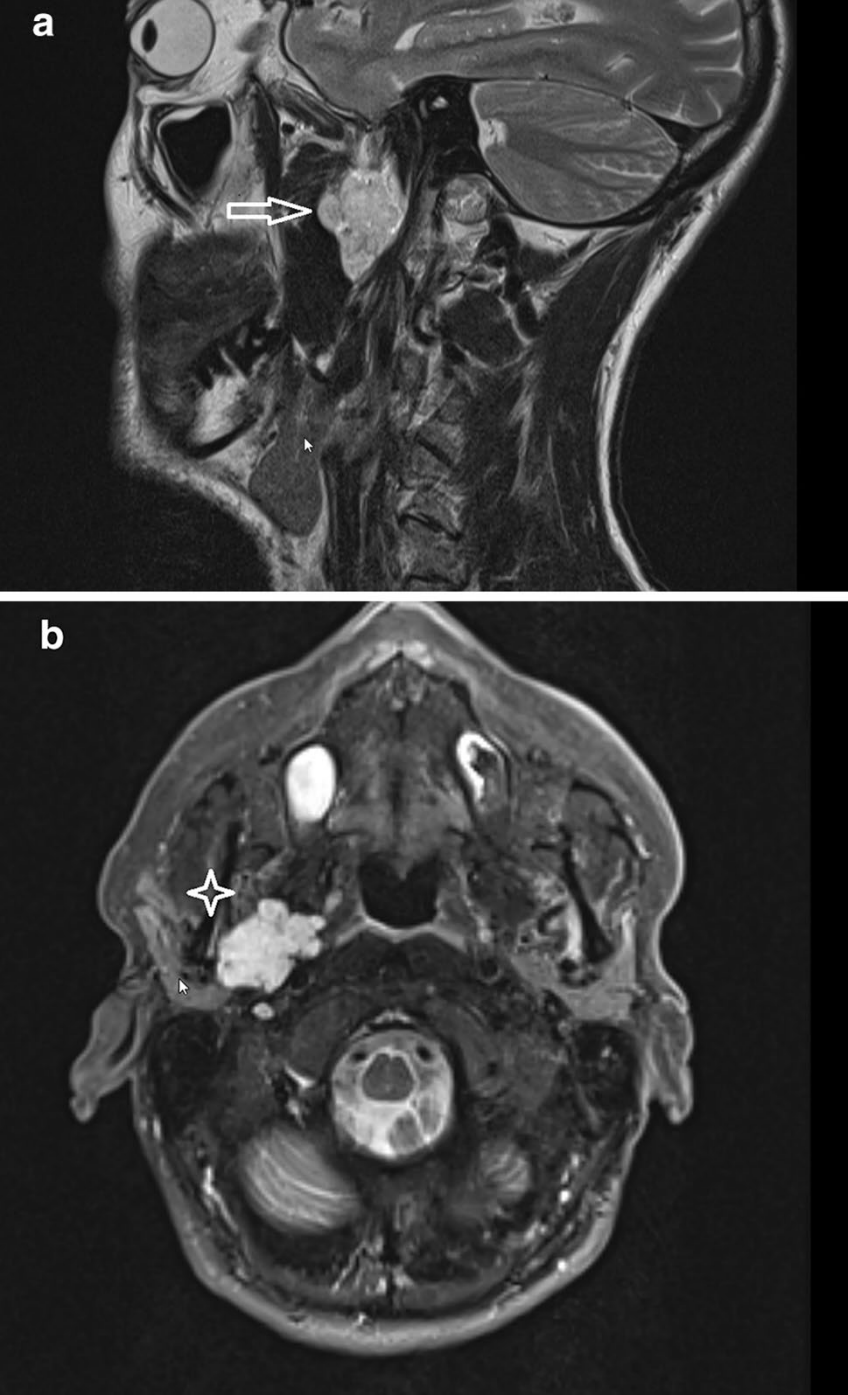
a Parasagittal MRI view of a parapharyngeal pleomorphic adenoma (arrow) (23 × 31 mm; T2). b Axial MRI view of a parapharyngeal pleomorphic adenoma (cross) (17 × 24 mm; T2)
